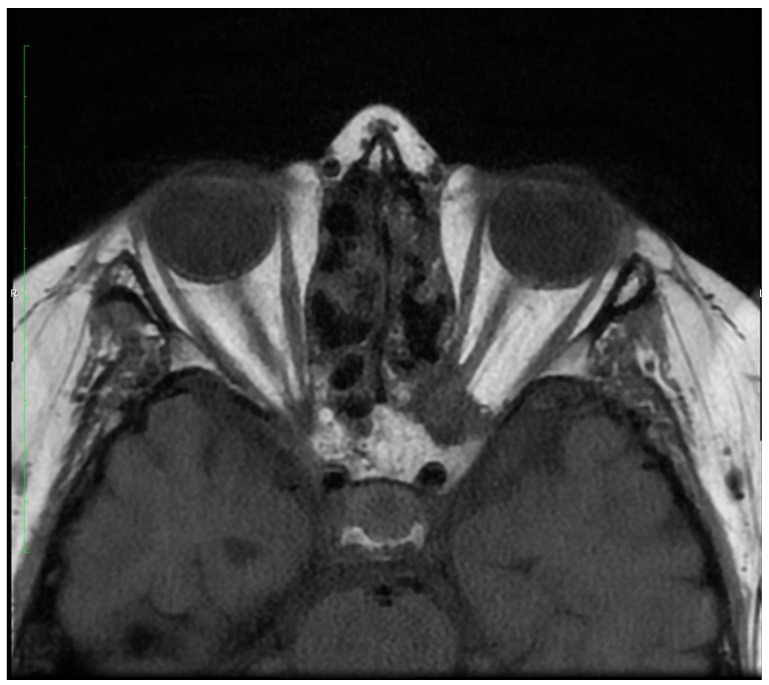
MRI of axial plane—a compressive mass in the left retrobulbar space causing the compression of the left optic nerve.
